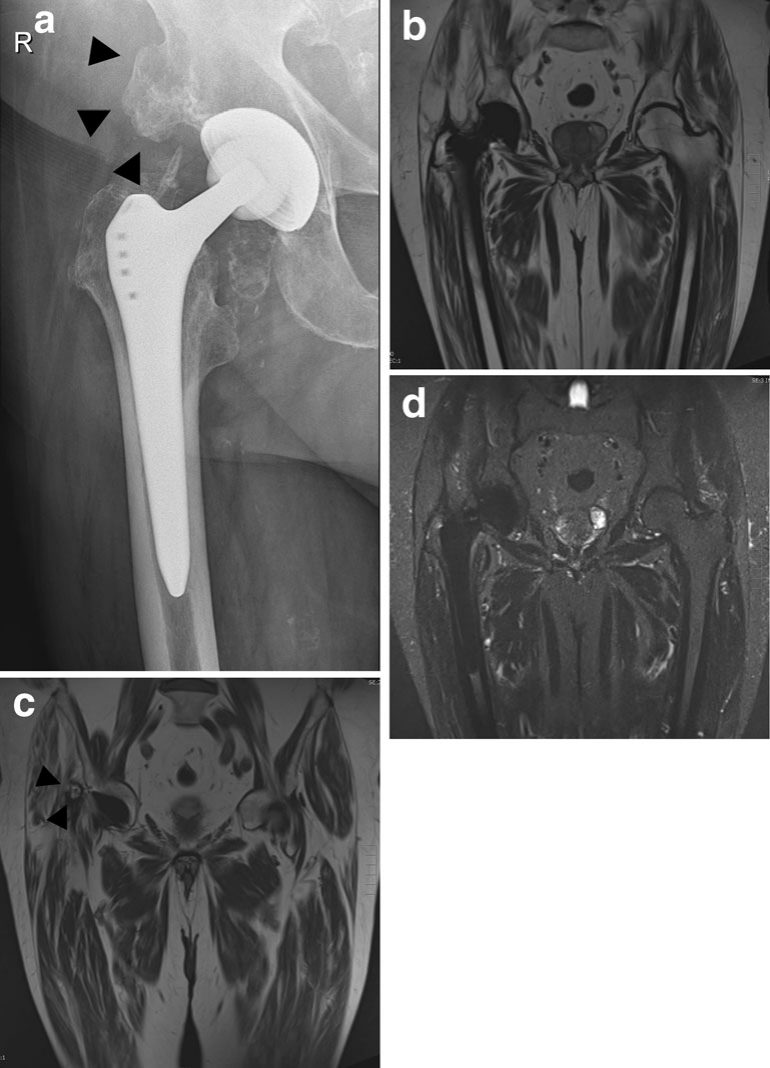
Although current state-of-the art MRI with Metal Artefact Reduction Sequences allows assessment of correct position of the hip prosthesis as well as periarticular abnormalities, mature heterotopic bone formation (*arrowheads* in *A* and *C*) is often more readily visible on plain radiographs than on MRI due to similar signal of mature bone marrow and fatty infiltration within the gluteus musculature at the site of the hip prothesis. a AP radiograph. Cementless total hip arthroplasty. Heterotopic bone formation (*arrowheads*), 7 years postoperatively. b T1-weighted, coronal image (WI) of the pelvis in the same patient. c T1-weighted, coronal image (WI) of the pelvis at a more anterior location barely showing heterotopic bone formation (*arrowheads*). d STIR, coronal image of the pelvis in the same patient
